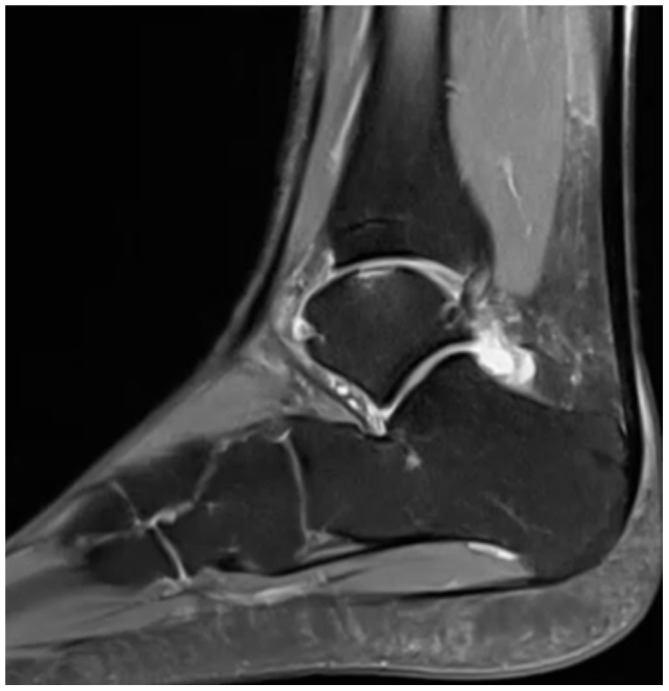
MRI of a lateral osteochondral lesion of the talus.
